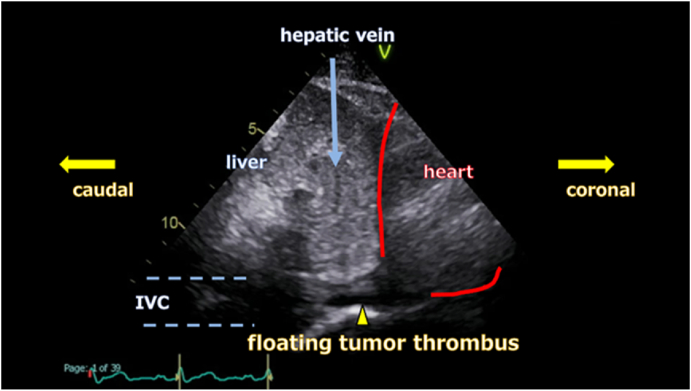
Abdominal ultrasonography The IVC region invaded by the tumor thrombus (yellow triangle) is located below the hepatic vein (blue arrow). The tumor thrombus above the hepatic vein is mobile and floating, while the IVC site where the tumor thrombus has invaded is nearly occluded, resulting in very low blood flow. IVC; inferior vena cava.
